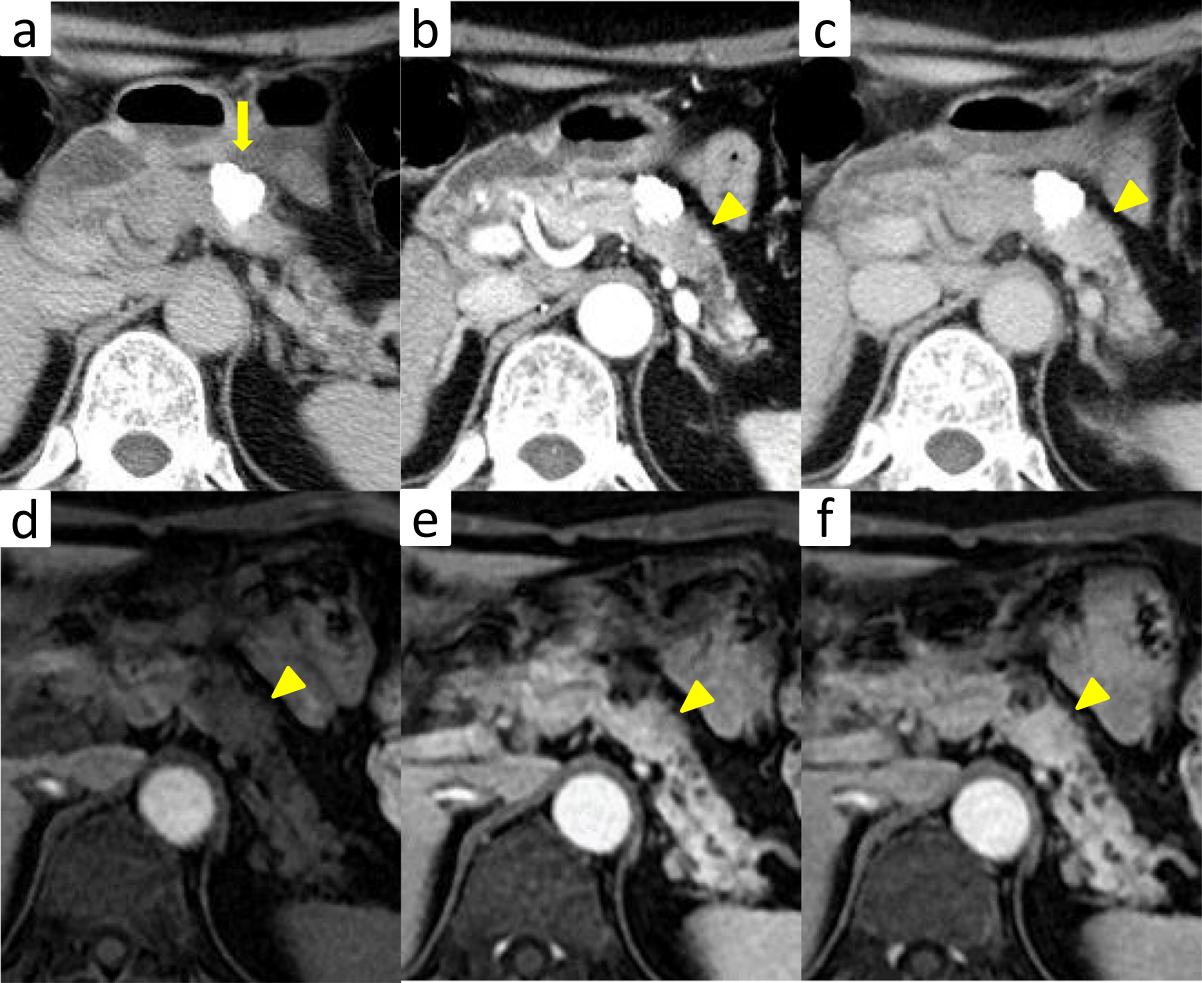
Plain CT, contrast-enhanced CT, and Gd-EOB-DPTA-enhanced MRI. Plain CT showing a calcification on the head side of the nodule (yellow arrowhead). Contrast-enhanced CT and Gd-EOB-DPTA-enhanced MRI showing a 21-mm nodule that showed gradually intense enhancement in the pancreatic body (yellow arrow). a Plain CT. b Arterial phase of CT. c Equilibrium phase. d Pre-enhancement phase of MRI. e Early phase of MRI. f Late phase of MRI. CT, computed tomography; Gd-EOB-DPTA, gadolinium-ethoxybenzyl-diethylenetriamine; MRI, magnetic resonance imaging
